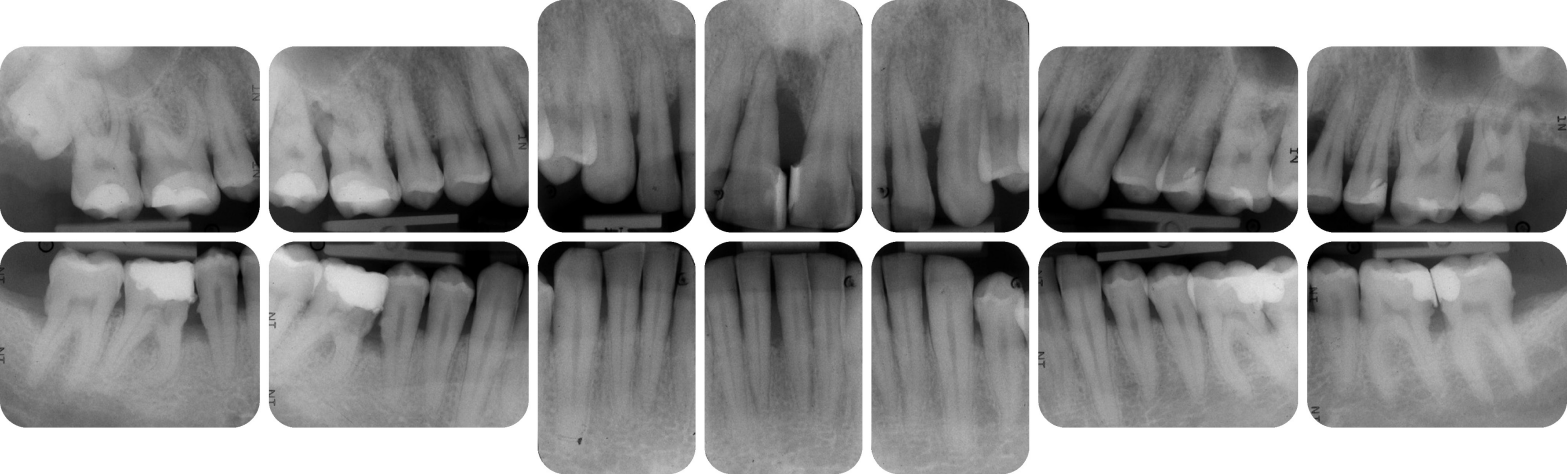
Radiographic images at baseline (ie, before steps 1 and 2 of periodontal therapy)
